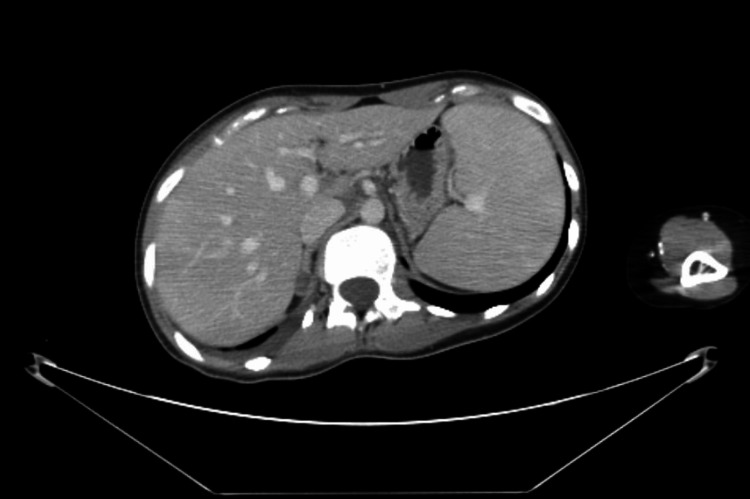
Computed tomography of the abdomen/pelvis with contrast: mild hepatomegaly, moderate splenomegaly, and a nondistended gallbladder.
